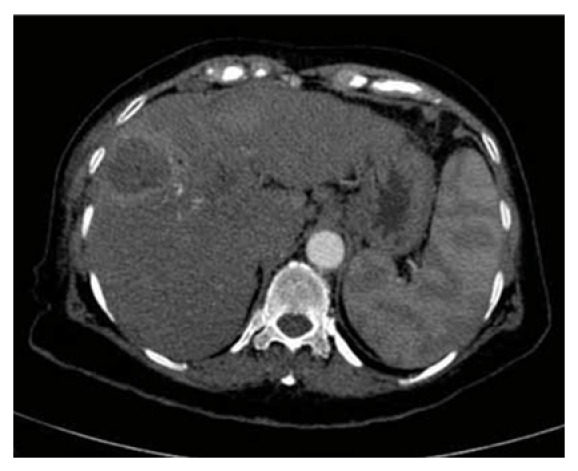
A 70-year-old woman with two lesions of hepatocellular carcinoma. Contrast-enhanced CT scan shows two hepatocellular masses in segment V (treated before with RFA) and II, under the ribs.
